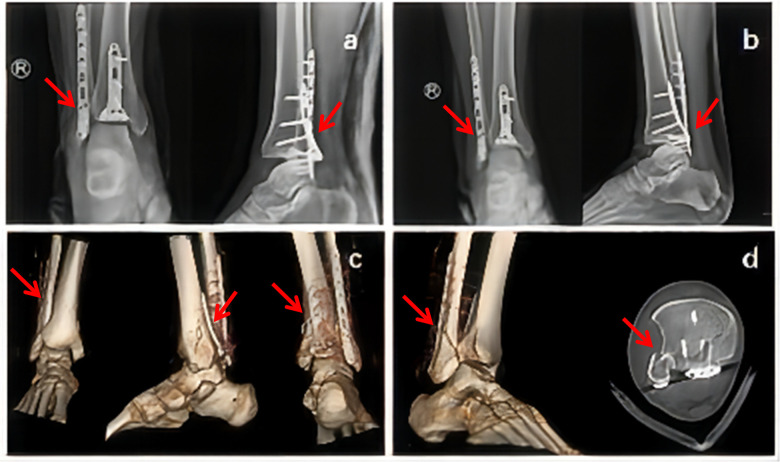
(a) The first review of x-ray after operation showed that the anterior space of the ankle joint is too large; (b) the x-ray of the post-operative dorsal flexion cast fixation showed that the anterior gap of the ankle joint decreases, but the ankle point still showed poor result; (c) postoperative review of CT plus three-dimensional reconstruction, Bosworth fracture-dislocation was not corrected.
